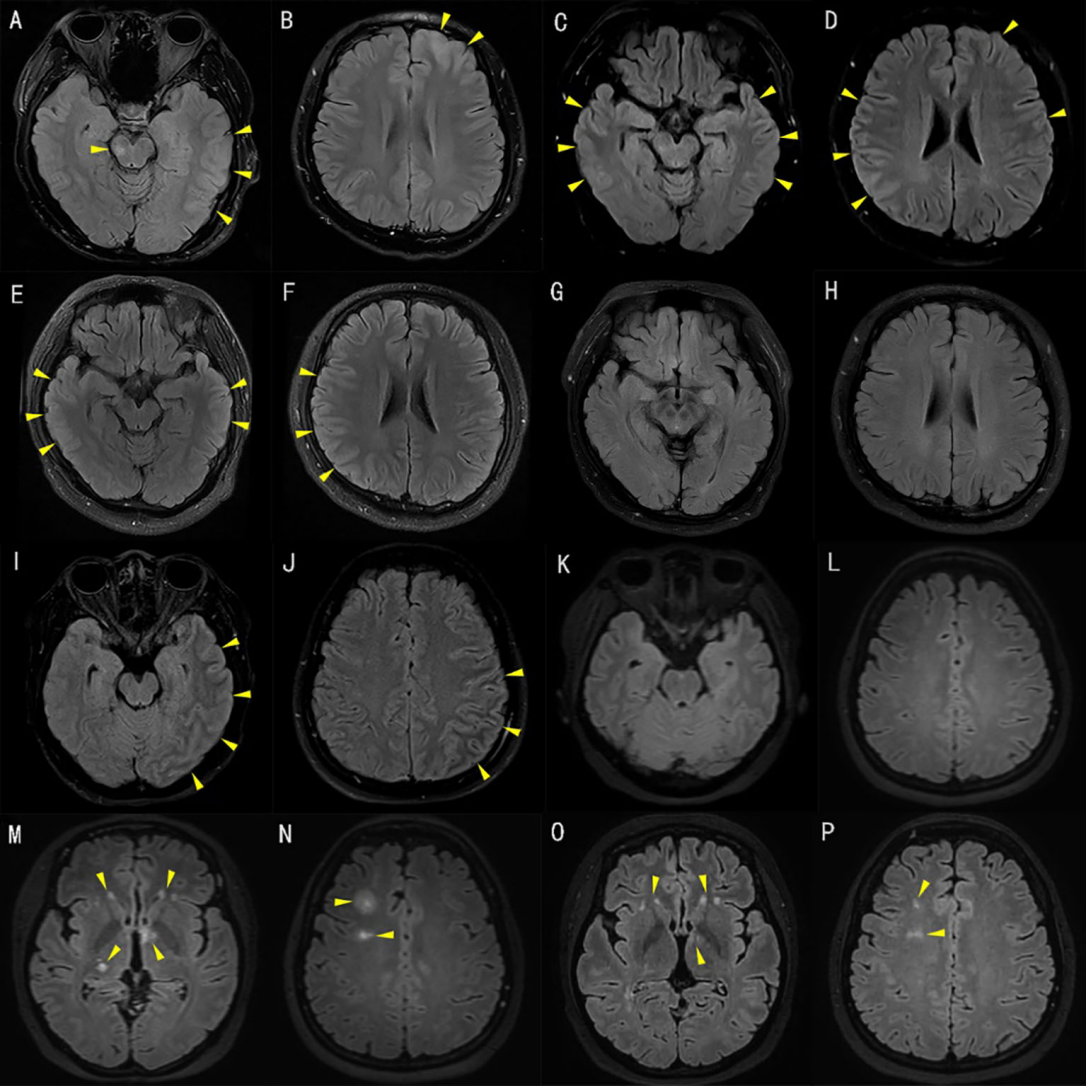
T2 fluid-attenuated inversion recovery (FLAIR) of brain magnetic resonance imaging (MRI). [A–H patient 1, I–P patient 2]. T2 FLAIR brain MRI of patient 1 at initial presentation demonstrated hyperintensities involving the brainstem, left frontal, parietal, and temporal cortex (A, B). T2 FLAIR brain MRI after admission to our hospital and suffering from status epilepticus demonstrated hyperintensities involving the left frontal cortex and bilateral temporo-parietal cortex (C, D). Repeat T2 FLAIR brain MRI three weeks after immunotherapy initiation showed improvement in the bilateral temporo-parietal cortex (E, F). There were no obvious lesions on images seven months after the initial treatment (G, H). Axial T2 FLAIR brain MRI of patient 2 at initial presentation demonstrated hyperintensities involving the left temporal, parietal, and occipital cortex (I, J). T2 FLAIR brain MRI ten days after immunotherapy initiation was normal (K, L). T2 FLAIR brain MRI after disease relapse demonstrated multiple hyperintensities, in keeping with acute disseminated encephalomyelitis involving the bilateral thalamus, basal ganglia, and right centrum semiovale (M, N). At re-assessment four months after second discharge, the patient was clinically asymptomatic with improving FLAIR lesions on repeat T2 FLAIR brain MRI (O, P).
